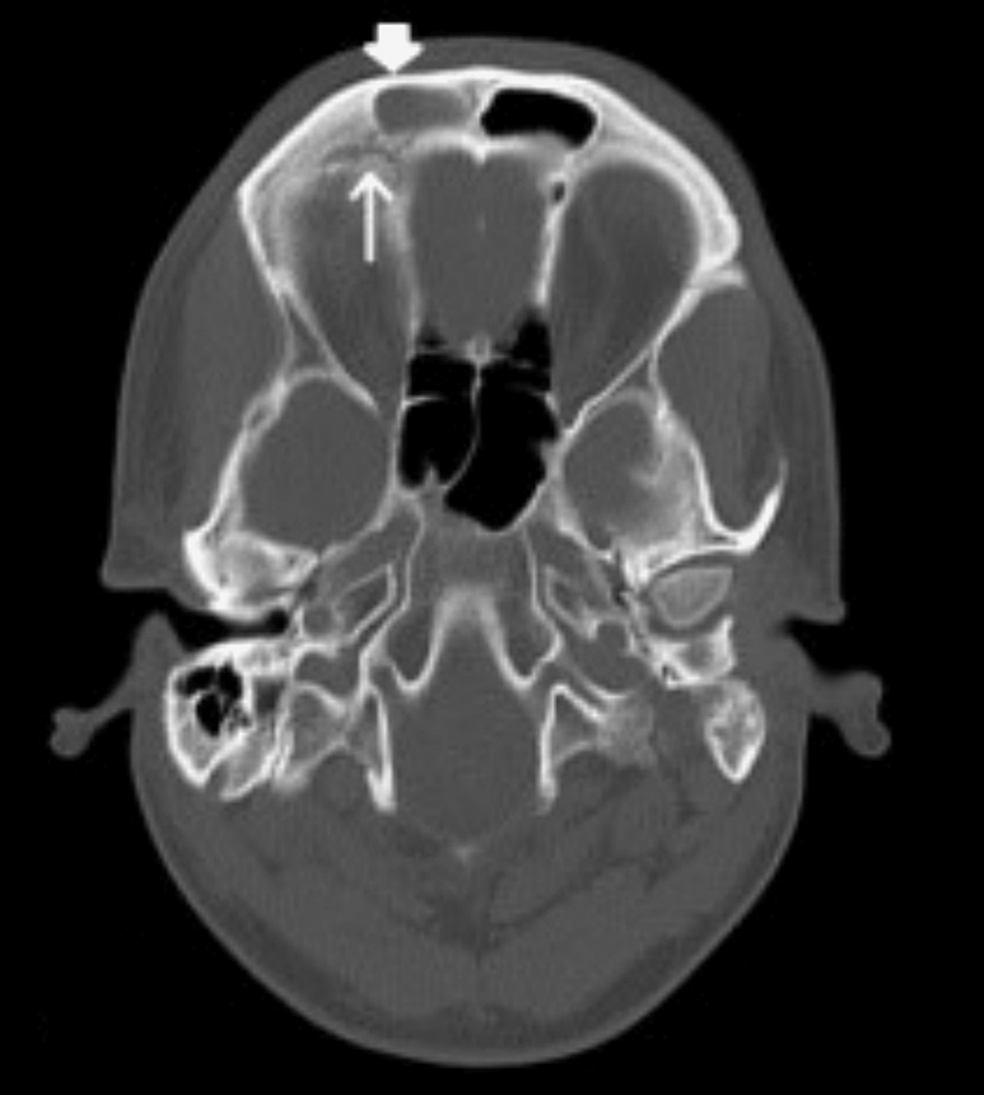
Axial CT without contrast demonstrating erosion of the posterior table of the right frontal sinus (arrow) and right frontal sinus opacification (arrowhead).
